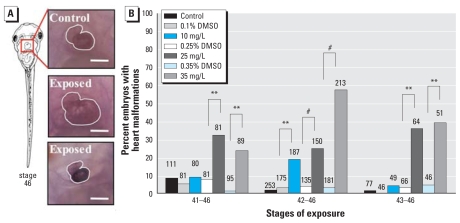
Incidence of heart malformations. (*A*) Ventral views of normal NF stage 46 heart morphology (top) in an untreated-control tadpole, and hypertrophic (middle) and reduced (bottom) hearts in age-matched tadpoles exposed to 35 mg/L atrazine (DMSO) from NF stage 41–46. Hearts were visualized using the CT-3 antibody and are outlined in white. Bars = 0.2 mm. (*B*) Incidence of reduced and enlarged hearts in tadpoles exposed to 10, 25, or 35 mg/L atrazine or vehicle (DMSO) from NF stage 41, 42, or 43 through NF stage 46. Sample sizes are indicated above bars. *p* < 0.001, and ^#^*p* < 0.0001, as determined by post hoc pair-wise contrasts from logistic regression.
